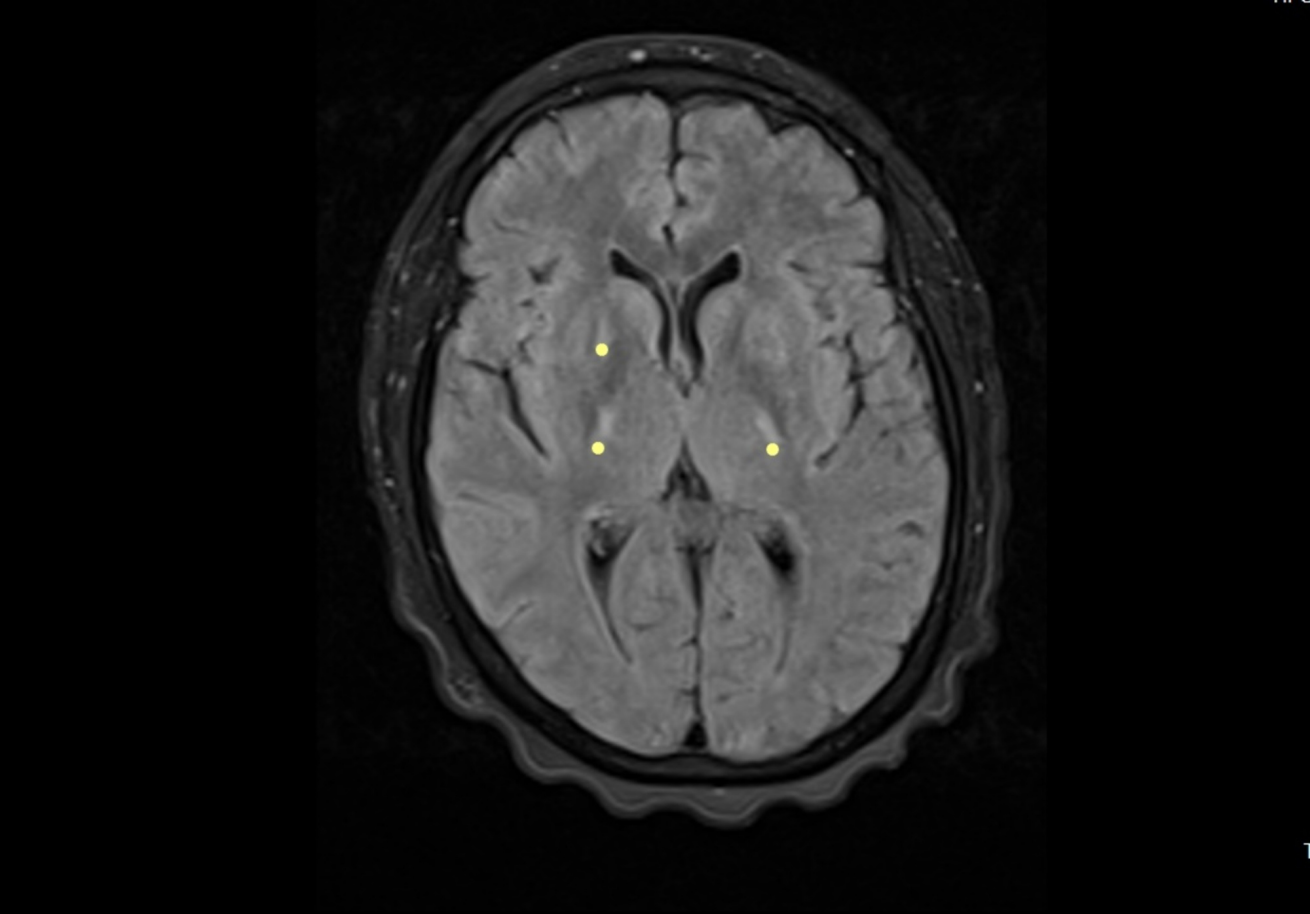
Brain MRI - view 1 FLAIR axial and T2-weighted coronal image. The image shows hyperintensity in the path of the corticospinal tract (yellow dots) MRI: magnetic resonance imaging; FLAIR: fluid attenuation inversion recovery
